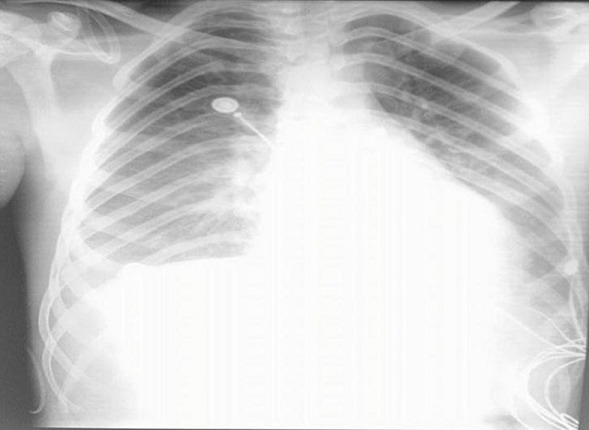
Chest X-ray showed pulmonary edema
